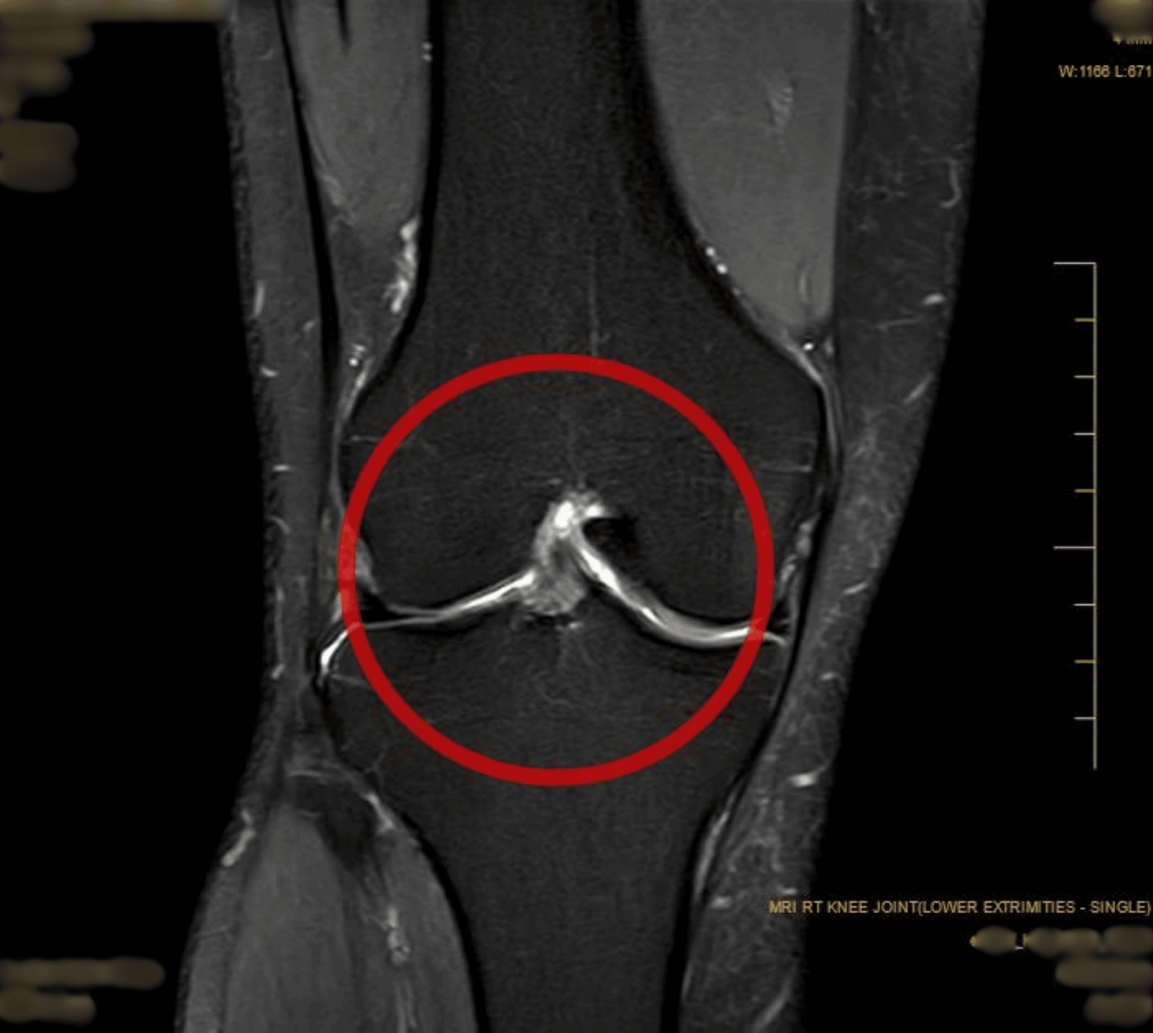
MRI of the right knee, coronal view, of a posterior cruciate ligament (PCL) ganglion cyst suggesting intrameniscal degeneration without a definitive tear.
